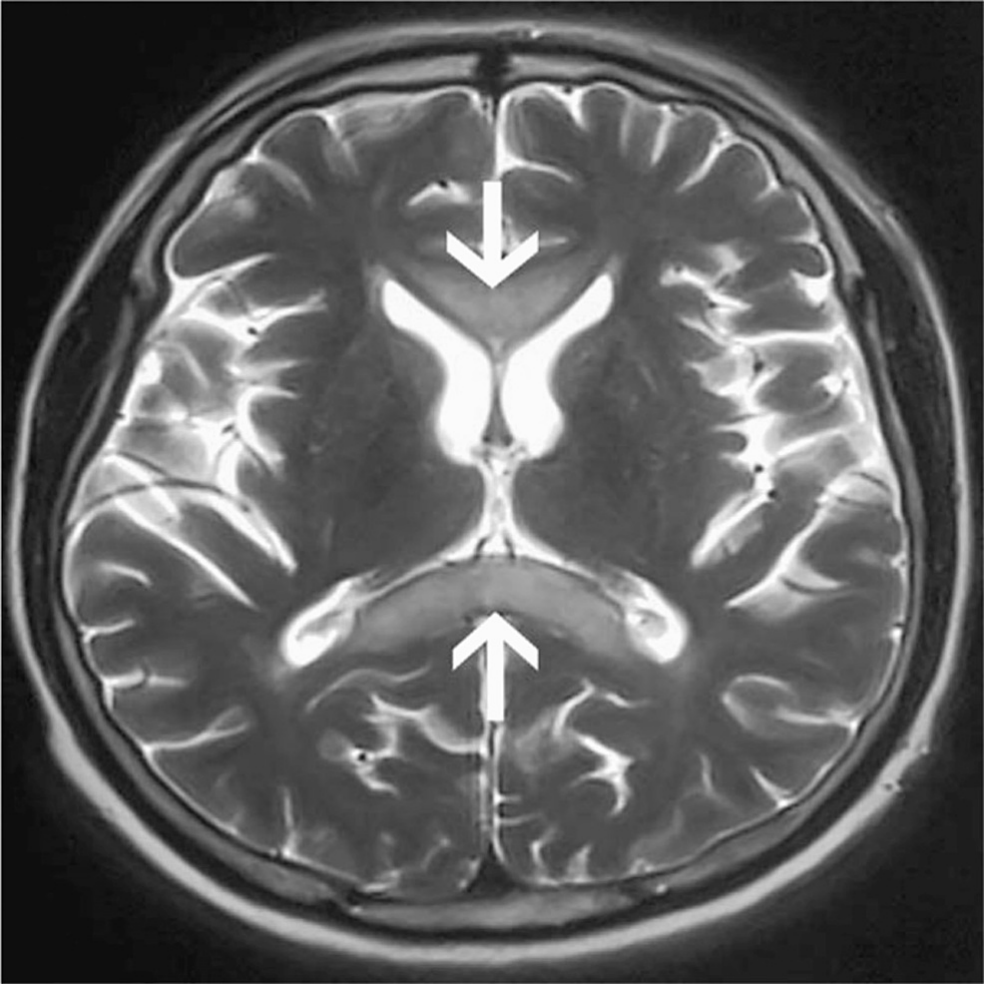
MRI T2 weighted Reference [30] is licensed under CC BY-NC 4.0
